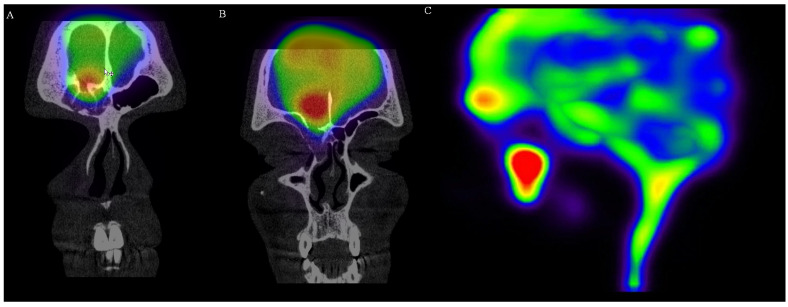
SPECT scanning: (A) coronal image of increased radioisotope activity over the right frontal sinus with bone defect; (B) coronal image of increased radioisotope activity over the anterior part cribriform plate with bone defect; (C) SPECT scan showing increased activity in the frontal area and the cumulation of radioisotope in the right maxillary sinus. (Images provided with the courtesy of the Institute on Nuclear and Molecular Medicine, Kosice.).
